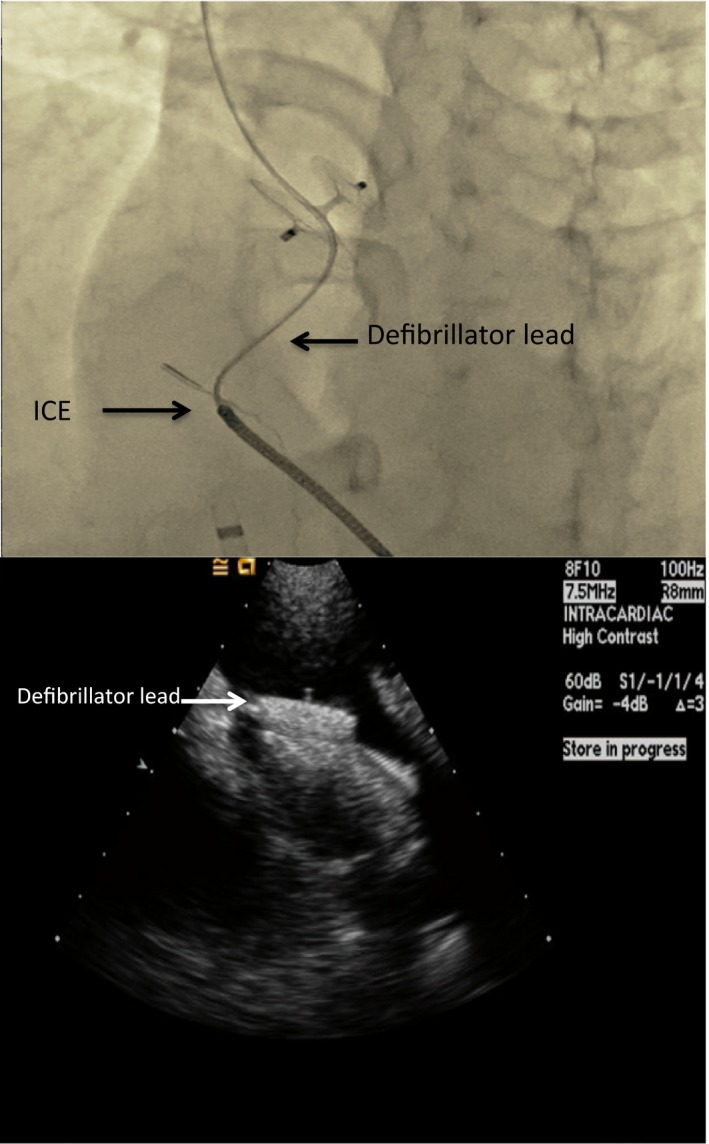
A, Fluoroscopic and (B) ICE images showing a trapped defibrillator lead in between the right atrial disc and the interatrial septum. ICE indicates intracardiac echocardiogram.
